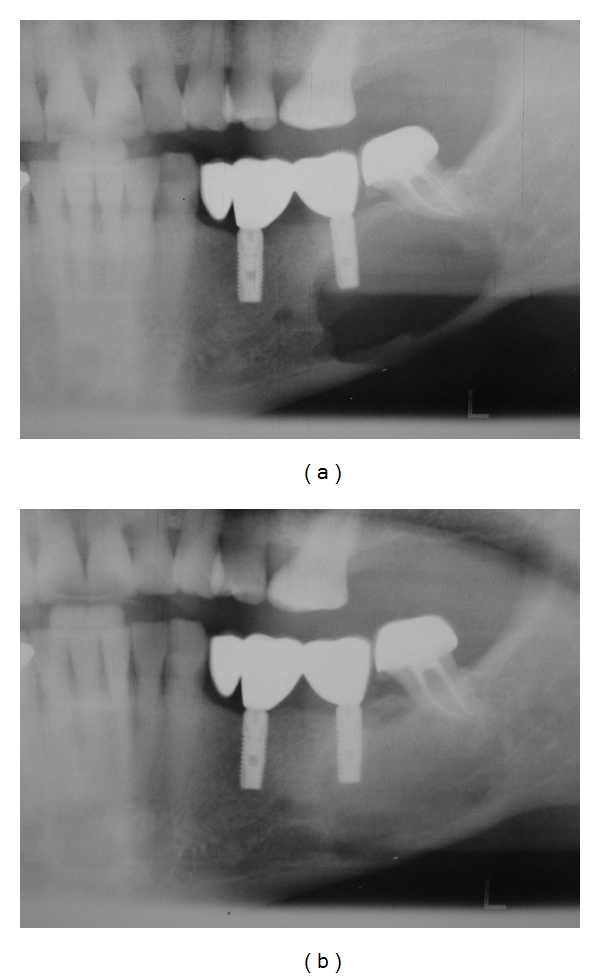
(a) Panoramic radiograph showed a large lytic lesion adjacent to the left implant and second left mandibular molar, diagnosed as MM (March 2007). (b) A new panoramic radiograph performed seven months after starting therapy revealed a complete regression of the mandibular lesion, supporting previous diagnosis of mandibular involvement of MM.
